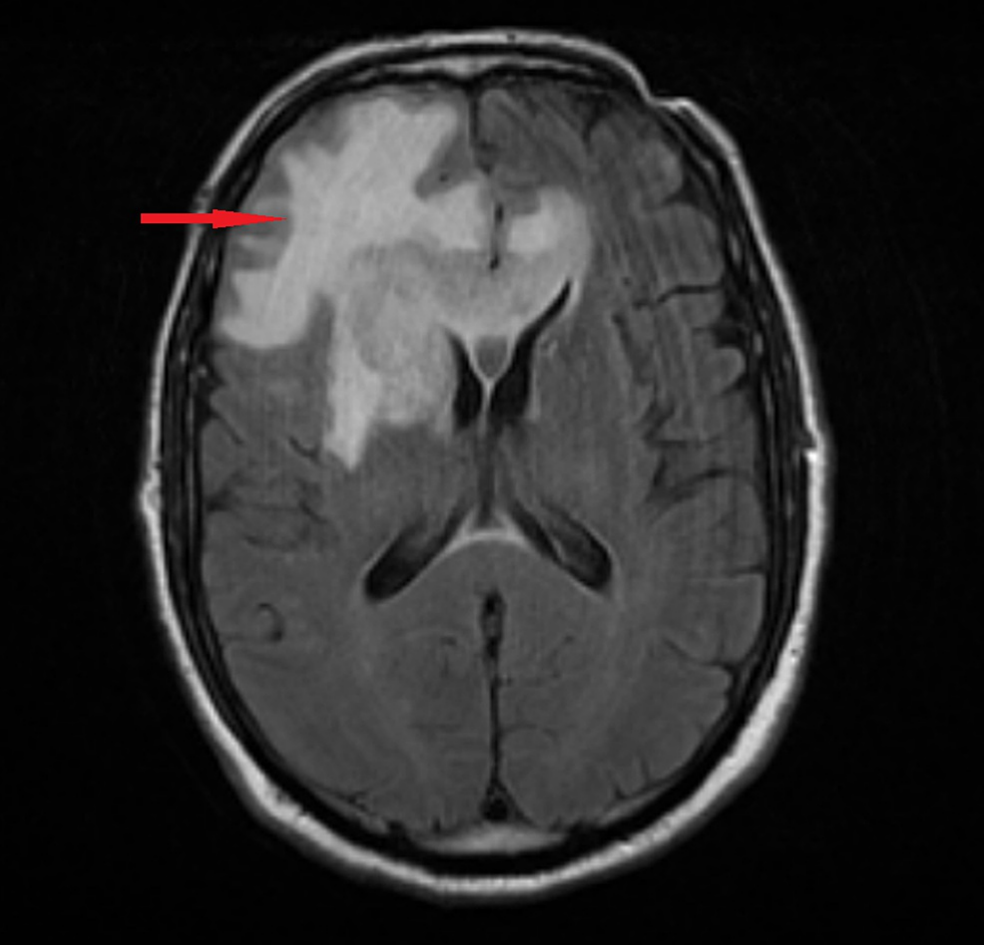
Enhancing intra-axial mass measuring 5.5 cm x 3.6 cm x 3.2 cm is predominantly located in the deep white matter of the right frontal lobe and also crosses midline (via the genu of the corpus callosum). Mass demonstrates DWI hyperintense signal, consistent with hypercellularity. DWI, diffusion-weighted imaging
